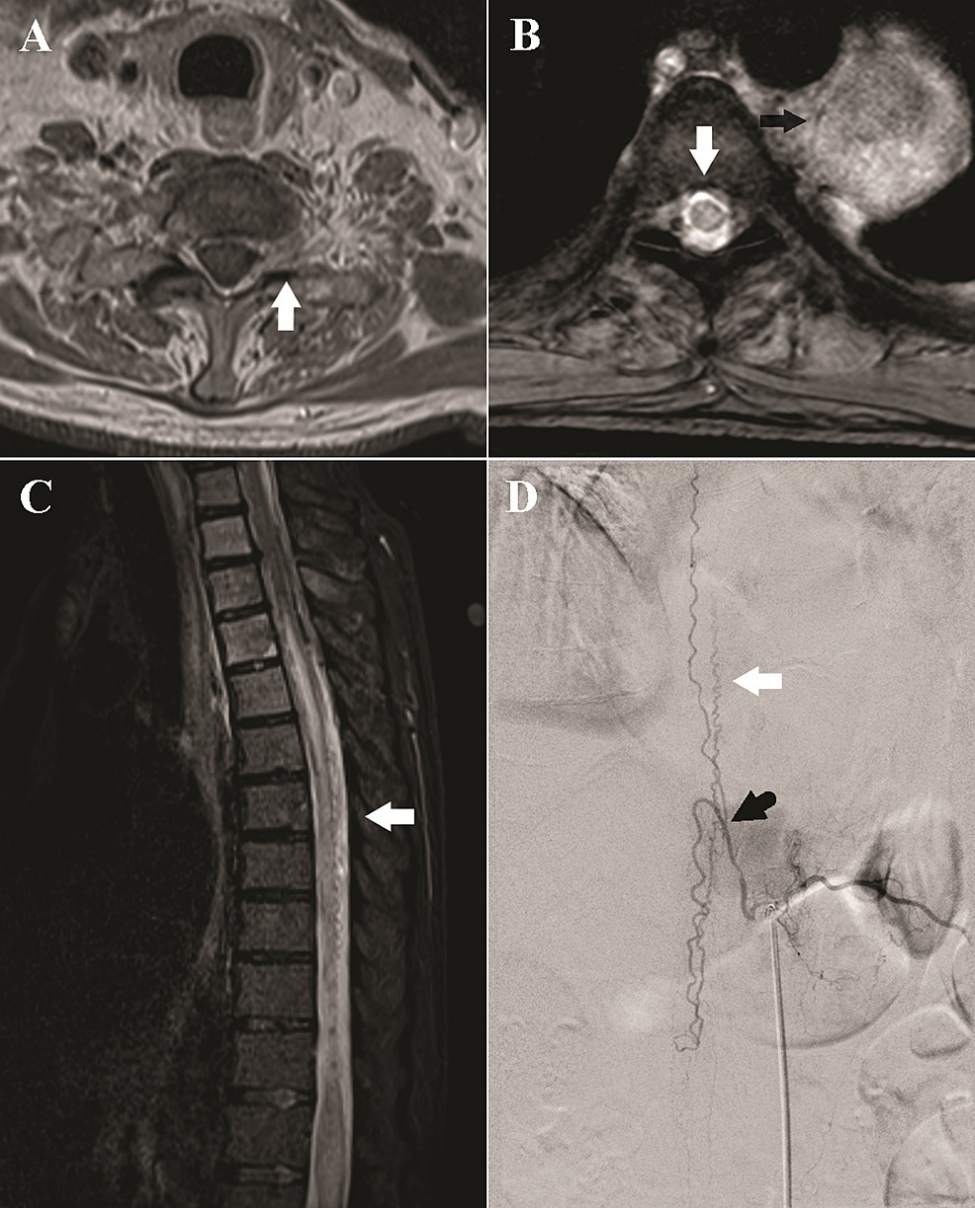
Thoracic Spine Imaging (A) Axial T1-weighted Gd+ images of the cervical spine at C8-T1 demonstrate the circumferential epidural enhancement and widening of the left neuroforamen (white arrow), concerning the metastatic disease. (B) Axial multiple echo data image combination (MEDIC) image at T5-6 demonstrates the central cord signal (white arrow). A large mass is seen along with the left aspect of the vertebral column (black arrow). ​(C) Sagittal short T1 inversion recovery (STIR) sequence of the thoracic spine demonstrates longitudinally extensive cord signal from T4 to the conus medullaris, consistent with venous congestion. There are prominent flow voids along the posterior aspect of the cord in the lower thoracic spinal canal (white arrow). (D) Angiography of the left T11 segment intercostal artery demonstrates evidence of a dural arteriovenous fistula with engorgement of the radicular vein (black arrow), which subsequently drains into a dilated spinal vein cranially and caudally (white arrow), corresponding to the flow voids visualized on MRI.
